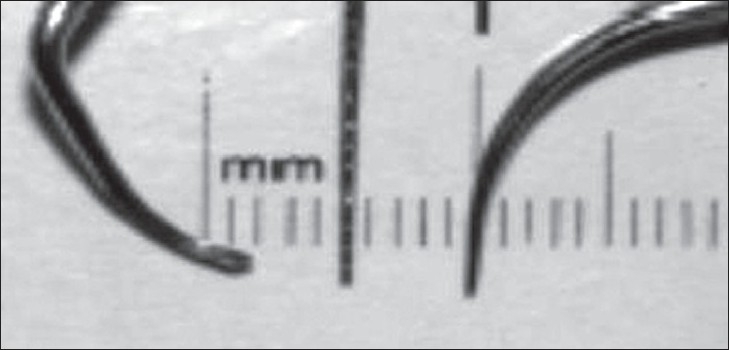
Scaler, probe and microultrasonic insert. (Kwan J Y; 2005)
